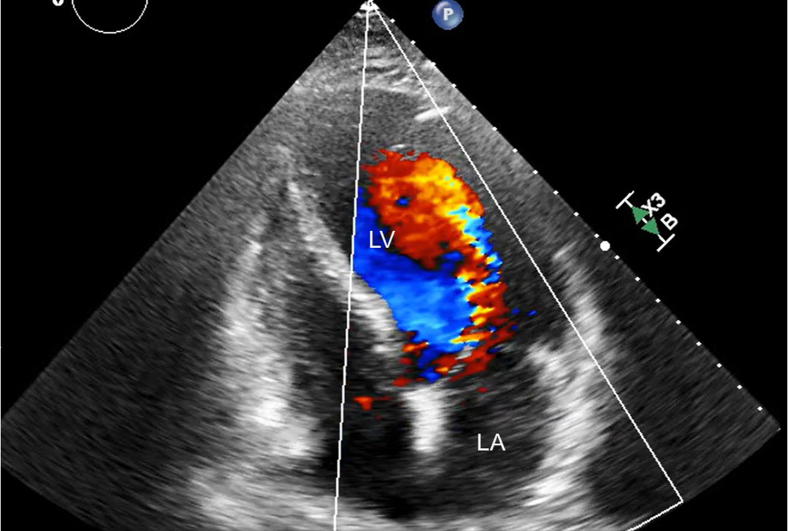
Transthoracic echocardiographic image showing severe aortic regurgitation. *LV*, Left ventricle; *LA*, left atrium.
